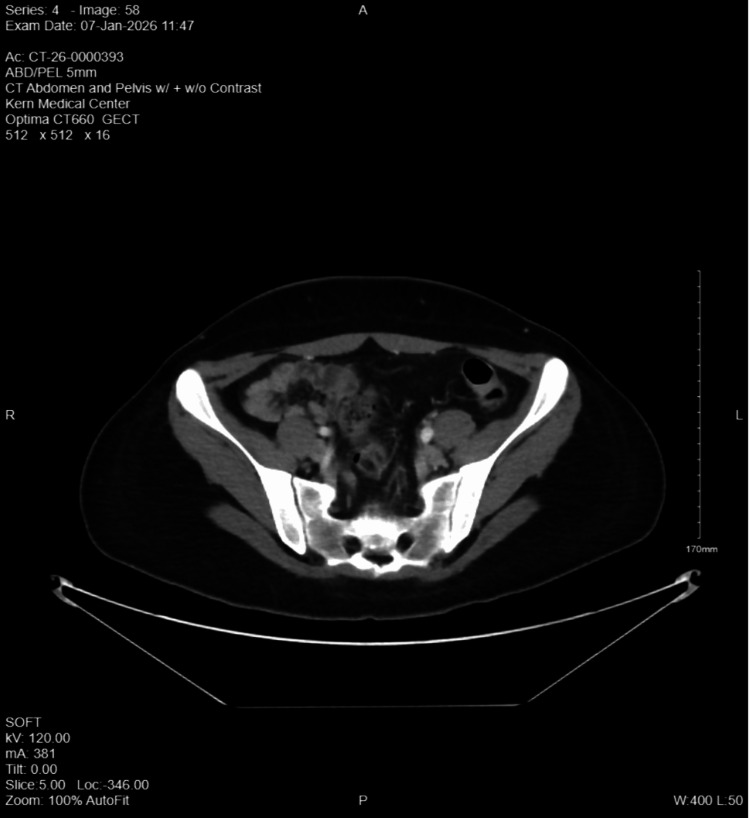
Follow-up CT scan of the abdomen and pelvis (January 7, 2026) demonstrating complete resolution with no evidence of a residual psoas abscess CT: computed tomography
